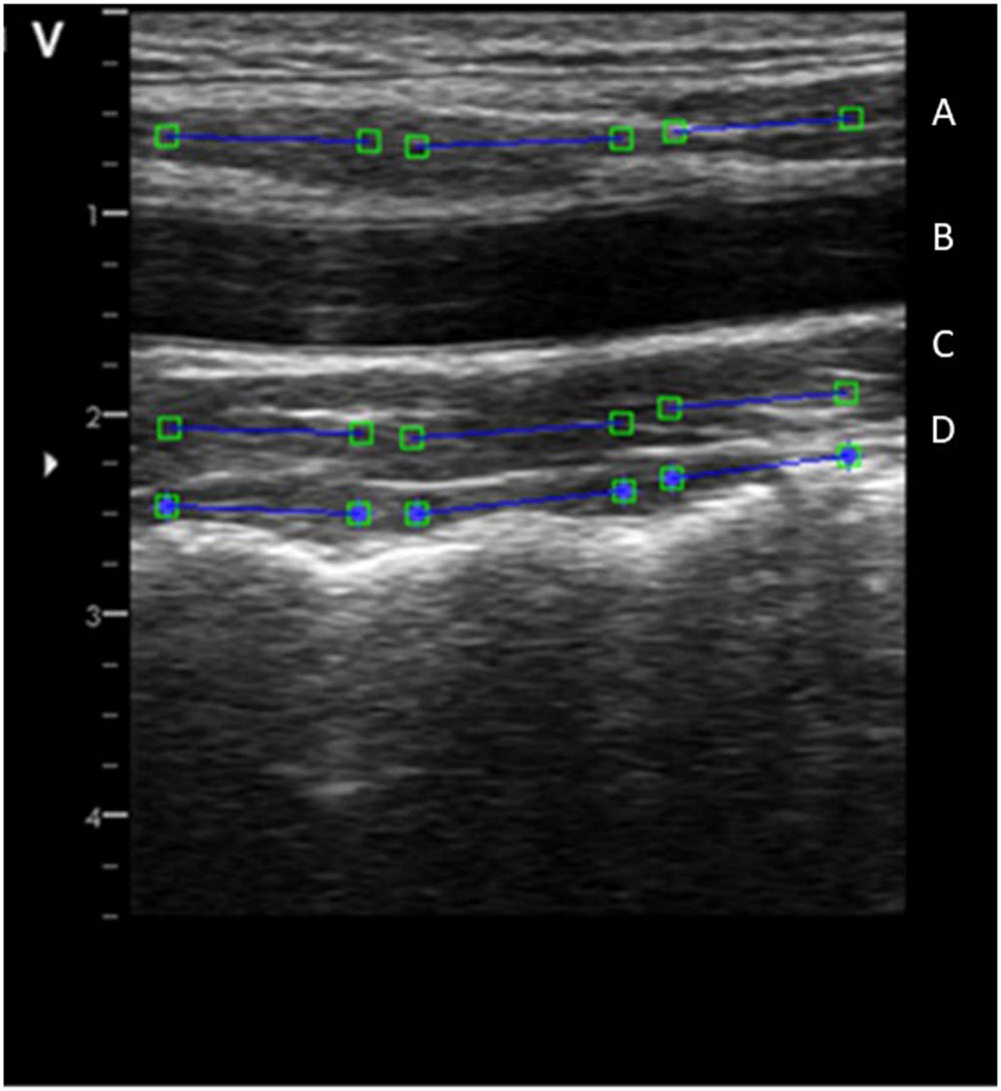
The ultrasound image shows the three range of interest (ROI) manually placed in the first frame of the video sequence of each muscle in longitudinal projection. The superficial sternocleidomastoid (A) shown at the top, followed by the common carotid artery (B), longus capitis (C) and longus colli (D). The ROI measuring the deformation (elongation and shortening) and the deformation rate (how fast the shortening and elongation occurs). Each ROI are indicated as a blue line with a square at each end.
